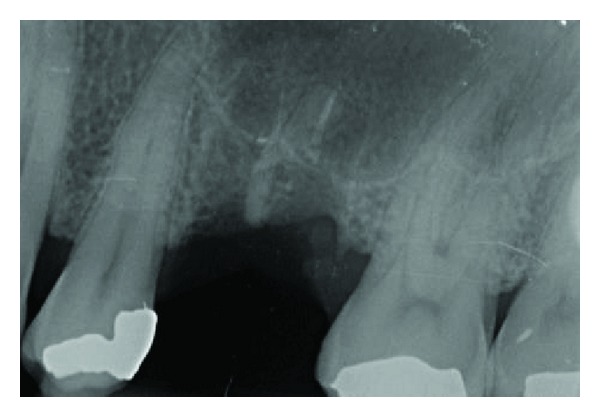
X-ray examination after first extraction: residual root and insufficient bony support.
